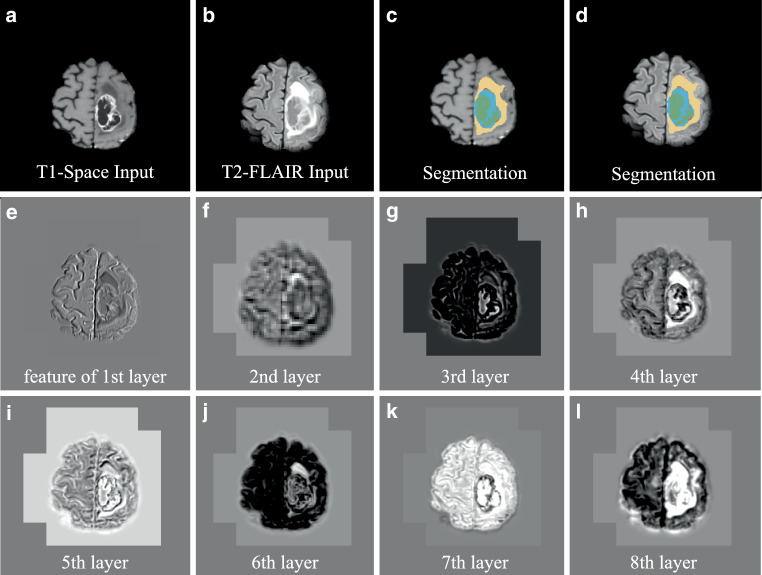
Some exemplary features extracted by DeepMedic [44] for glioma segmentation. The colors in the segmentation represent different tumor subregions: edema/invasion (*yellow*), nonenhancing tumor (*brown*), enhancing core (*cyan*), and necrotic core (*green*); a and b are T1-space and T2-FLAIR input images, respectively; c and d are the network segmentations overlaid with a and b, respectively; e–l are the first-channel features of the 1st to 8th convolutional layers in the first pathway of DeepMedic (displayed in an intensity window of $$[-1,1]$$)
